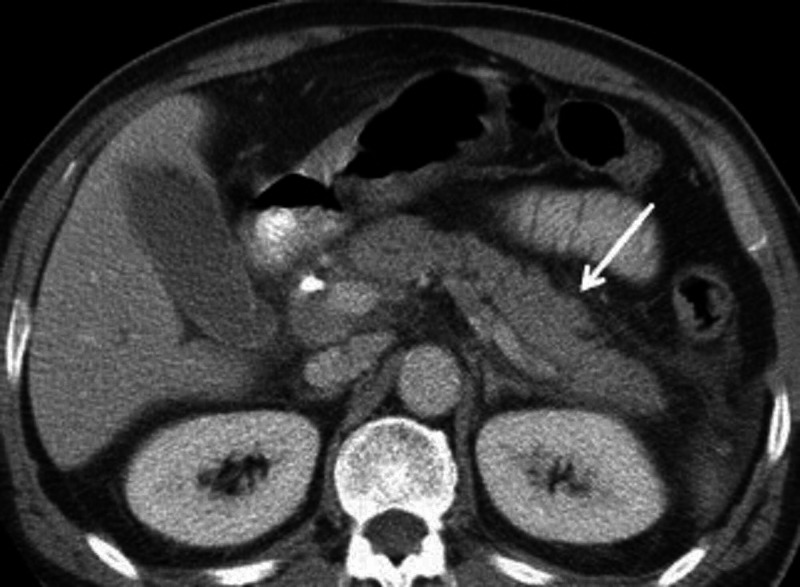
Abdominal CT scan showing interstitial edema of the pancreas with the homogenous enhancement of the pancreatic parenchyma and mild inﬂammatory changes (fat stranding)
